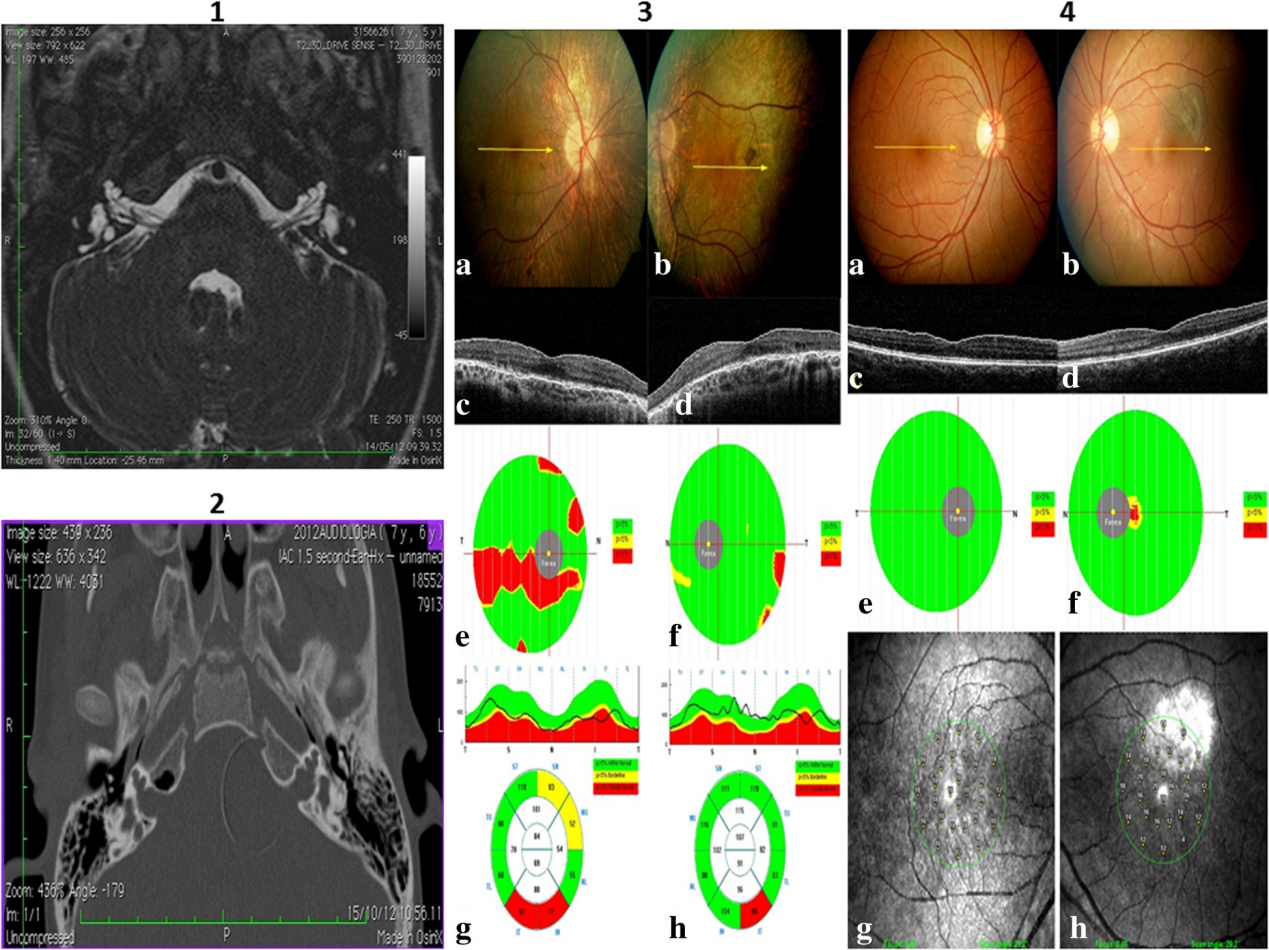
Images for subject IV-1: 1) Axial MR TSE drive T2-weighted image. Note the small bulbous dilatation of the lateral part of the IAC, with absence of the modiolus and of the bone at the fundus. The cochlea is dysmorphic and small in size. The vestibule is dilated bilaterally. Note also the dilatation of the vestibular acqueduct on the right side. 2) Axial CT. The absence of the bone fundi of IAC and of the modiolus are better depicted on CT. Ophthalmic results: 3) Male patient affected with choroideremia. Color fundus images (a, right eye; b, left eye) and cross sectional OCT B-scan (c, right eye; d, left eye) show diffuse atrophy of the retinal pigment epithelium and choriocapillaries and round retinal pigment changes at the posterior pole and midperiphery. Ganglion cell complex thickness shows a severe arciform thinning in (e) the right eye, while focal alterations are present in (f) the left eye. Retinal nerve fiber layer is reduced in the inferior quadrants in (g) the right and (h) left eye. 4) Female patient carrier of the choroideremia gene. Color fundus images (a, right eye; b, left eye), cross sectional OCT B-scan (c, right eye; d, left eye), ganglion cell complex thickness map (e, right eye; f, left eye) and microperimetry (g, right eye; h, left eye) are normal. A choroidal nevus is visible at the posterior pole in the left eye.
